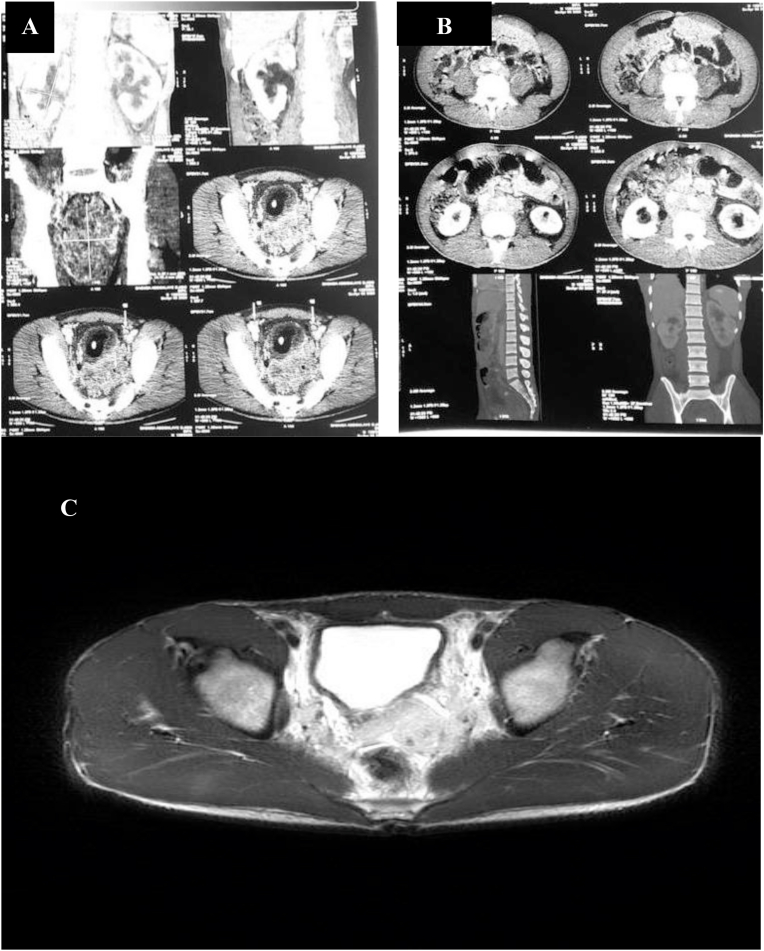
(A) Computed tomography (CT) scan of the abdomen and pelvis revealing a prostate tumor with bilateral ureterohydronephrosis and pelvic lymph node metastases (B) lumbo-aortic lymph node metastases and bone metastases (C) Pelvic Magnetic resonance imaging (MRI) showing a prostate tumor measuring 102× 82.6 × 64.4 mm with capsular crossing and invasion of the posterior bladder wall.
